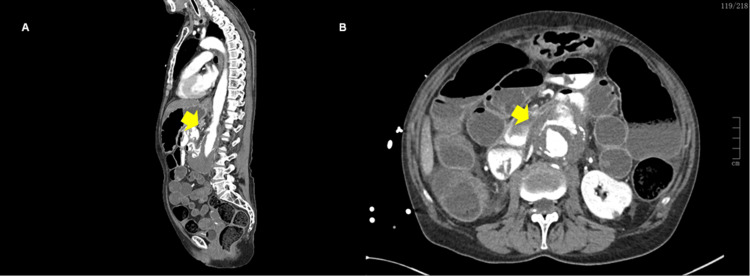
CTA demonstrating an AEF (A) Sagittal view showing extravasation of contrast from the abdominal aorta into the adjacent duodenum (yellow arrow). (B) Axial view confirming the communication between the aortic aneurysm and the duodenal lumen (yellow arrow). CTA: computed tomography angiography, AEF: aortoenteric fistula
